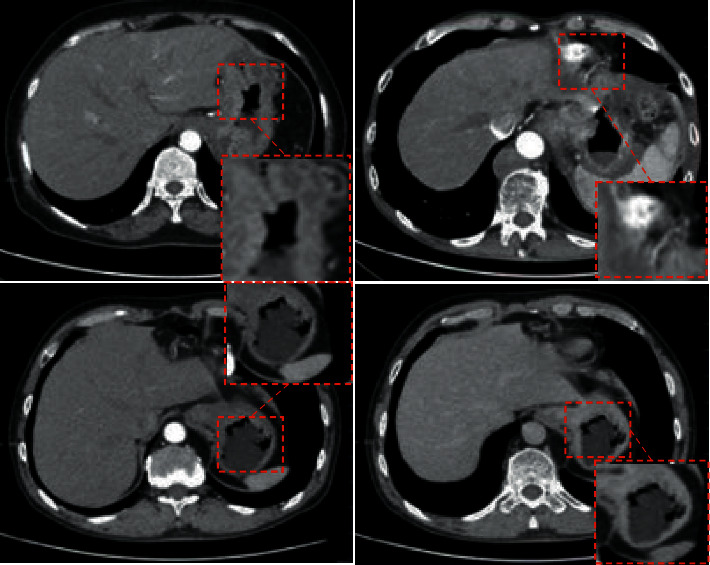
CT findings of four gastric cancer patients. The red marked area in the figure indicates
